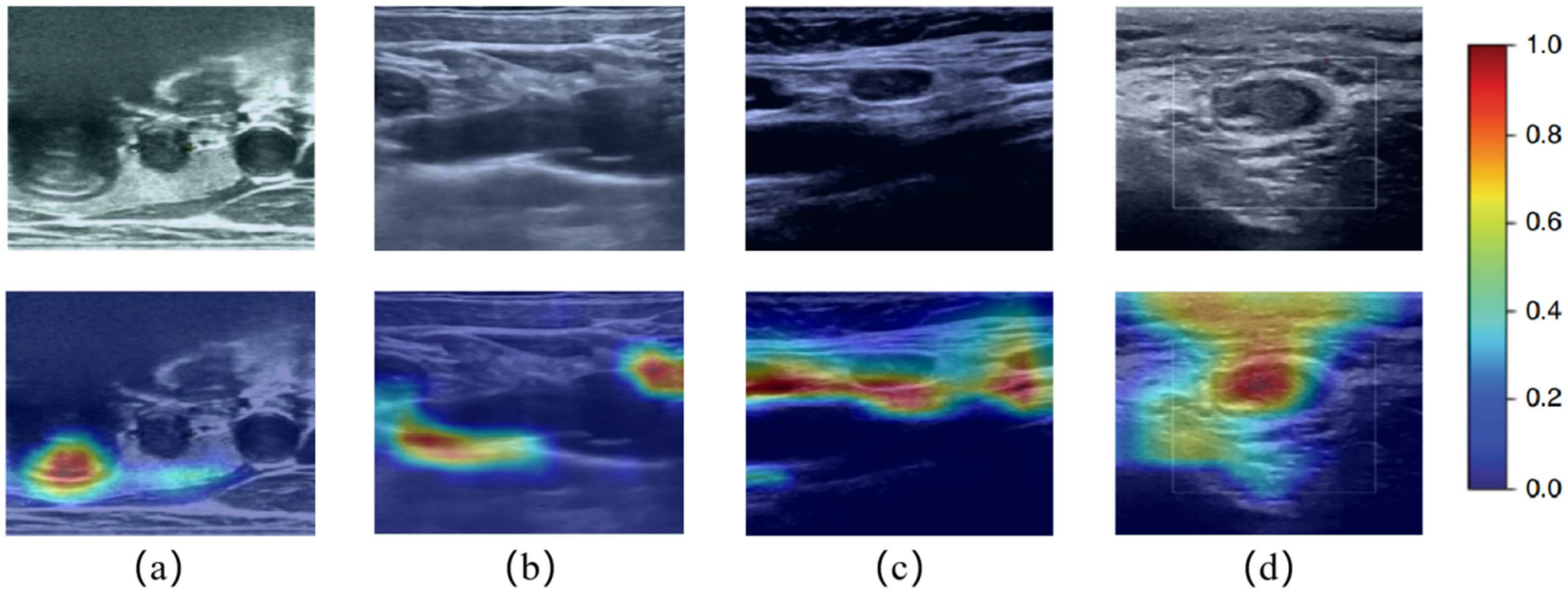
Ultrasound images and corresponding heatmaps of thyroid nodules. The heatmaps show the importance of the predictive image features of the AI architecture using different colors. Red and yellow represent the most powerful predictive areas of the tumor, and regions of blue and green show weaker predictive areas.
